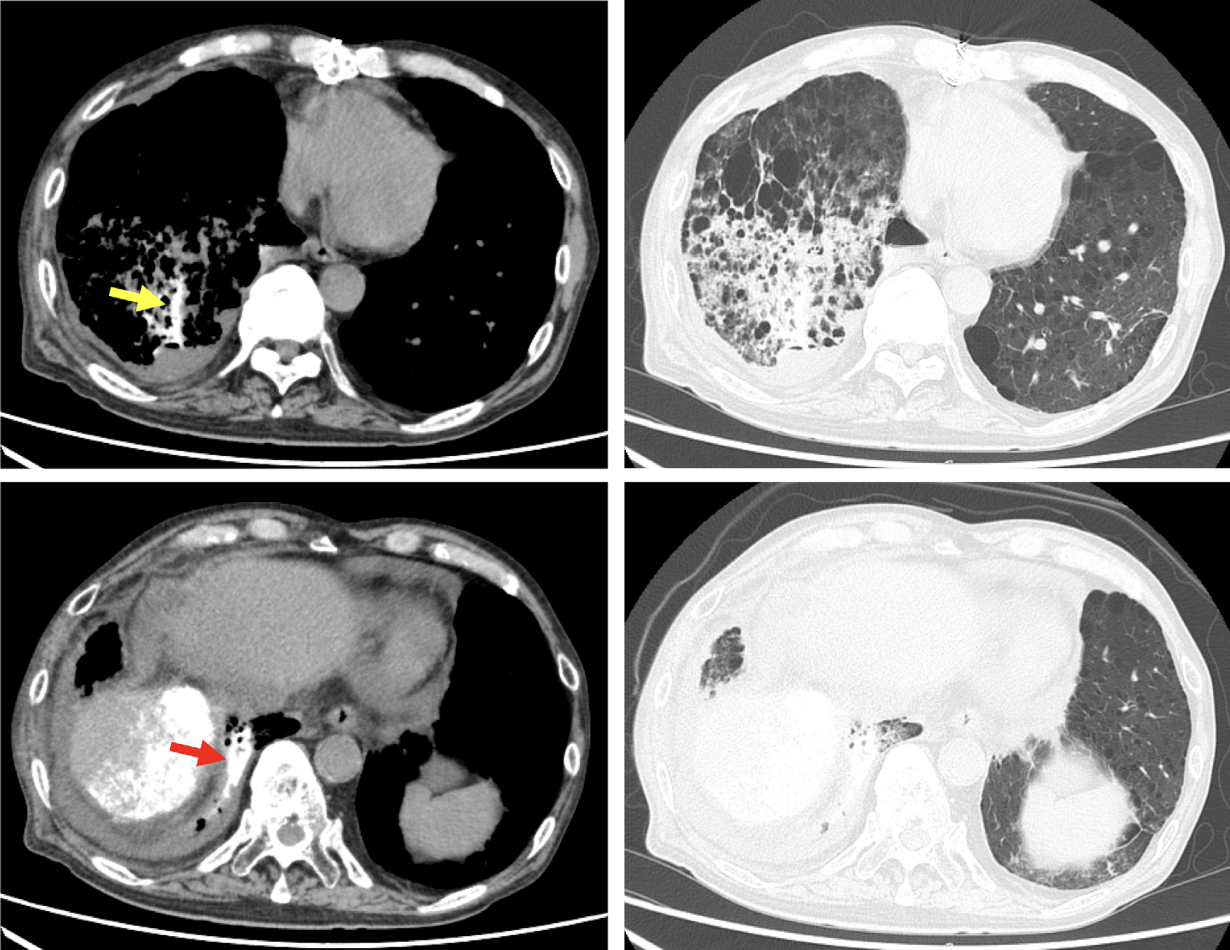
Consolidation was observed in the right lower lobe on non‐contrast computed tomography. A part of the consolidation (red arrow) and a pulmonary vein (V9, yellow arrow) had extremely high density.
